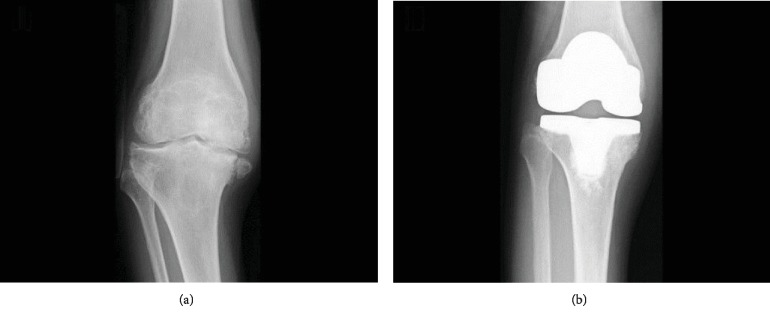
(a) Preoperative radiograph of the right knee showing mild varus. (b) Postoperative radiograph after right TKA.
